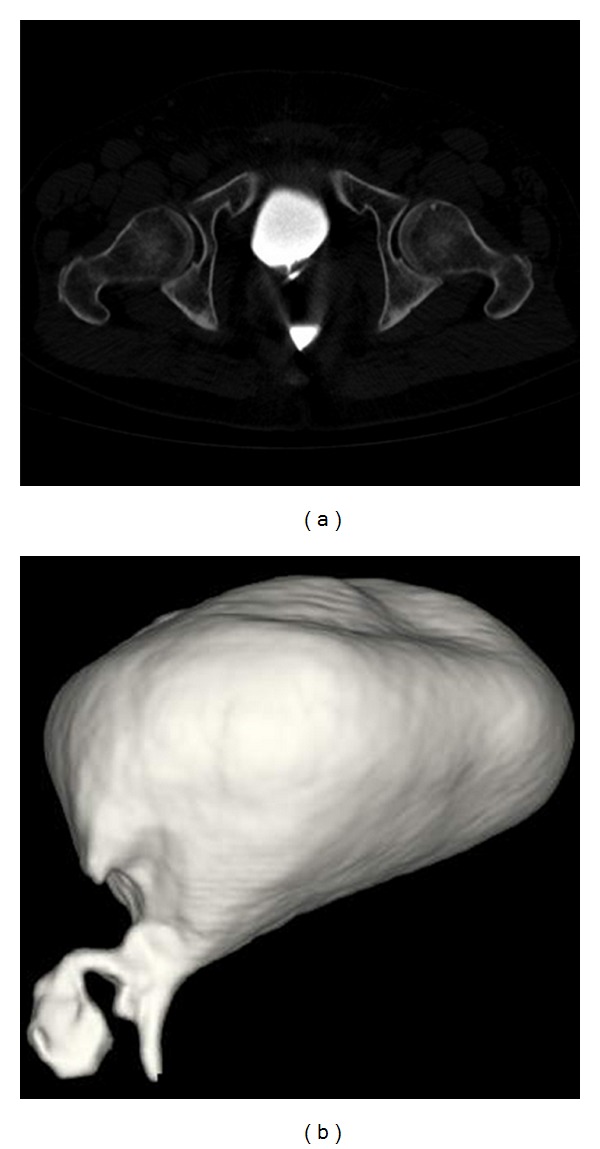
Pelvic CT scan with VR reconstruction. Pelvic CT scan was acquired before emptying the bladder of the contrast media, in order to confirm and to be sure that the bladder was not involved in the problem and that only the urethra was affected by the fistula.(a)Axial plane. (b)VR reconstruction.The latterallows to see very well the fistolous tract that connects the bladder to the rectum.
